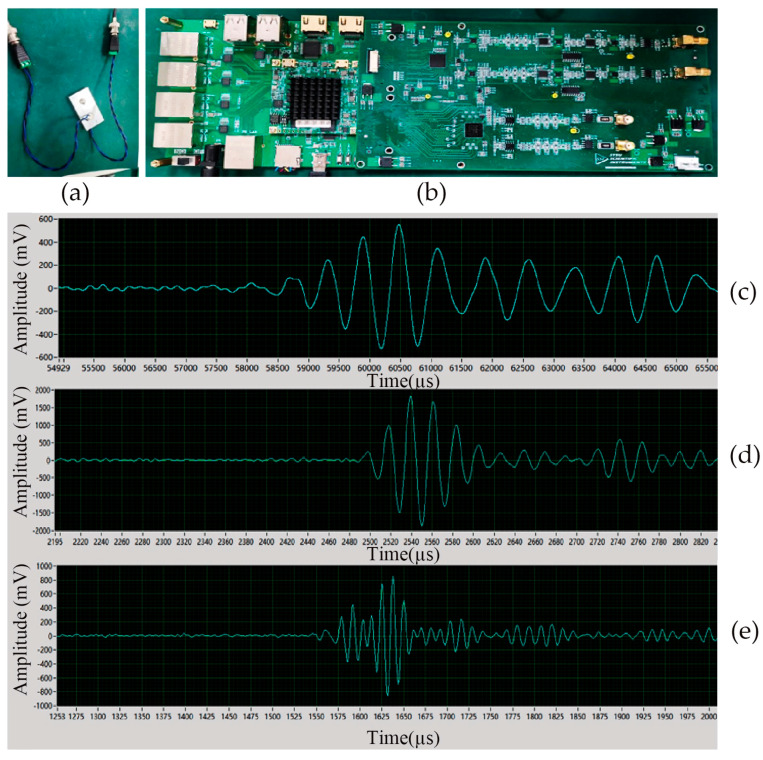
Experimental setup and echo images of ultrasonic testing using the upper computer: (a) Aluminum test sample. (b) Ultrasonic transmitter–receiver board. (c) Echo image collected for a 100 kHz five-peak wave input. (d) Echo image collected for a 300 kHz five-peak wave input. (e) Echo image collected for a 500 kHz five-peak wave input.
